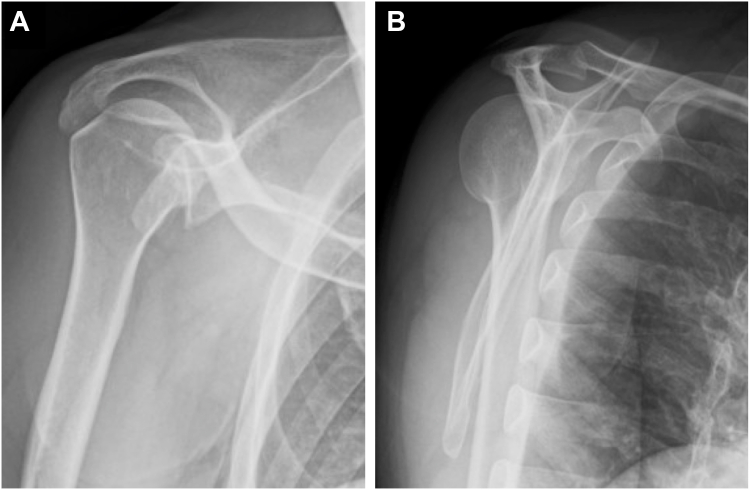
Initial radiographs of Case 2. (A) Anteroposterior view. (B) Scapula Y view.
